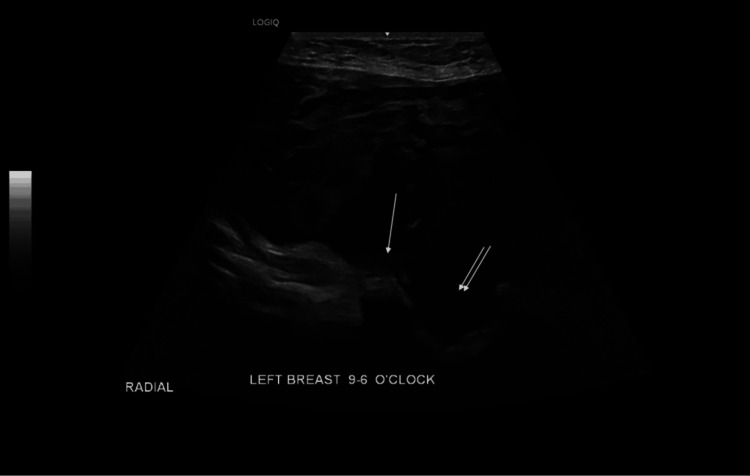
Left breast ultrasound showing mixed cystic and solid lesions The single arrow points at the solid component of the lesion, and the double arrows point at the cystic component of the lesion.
